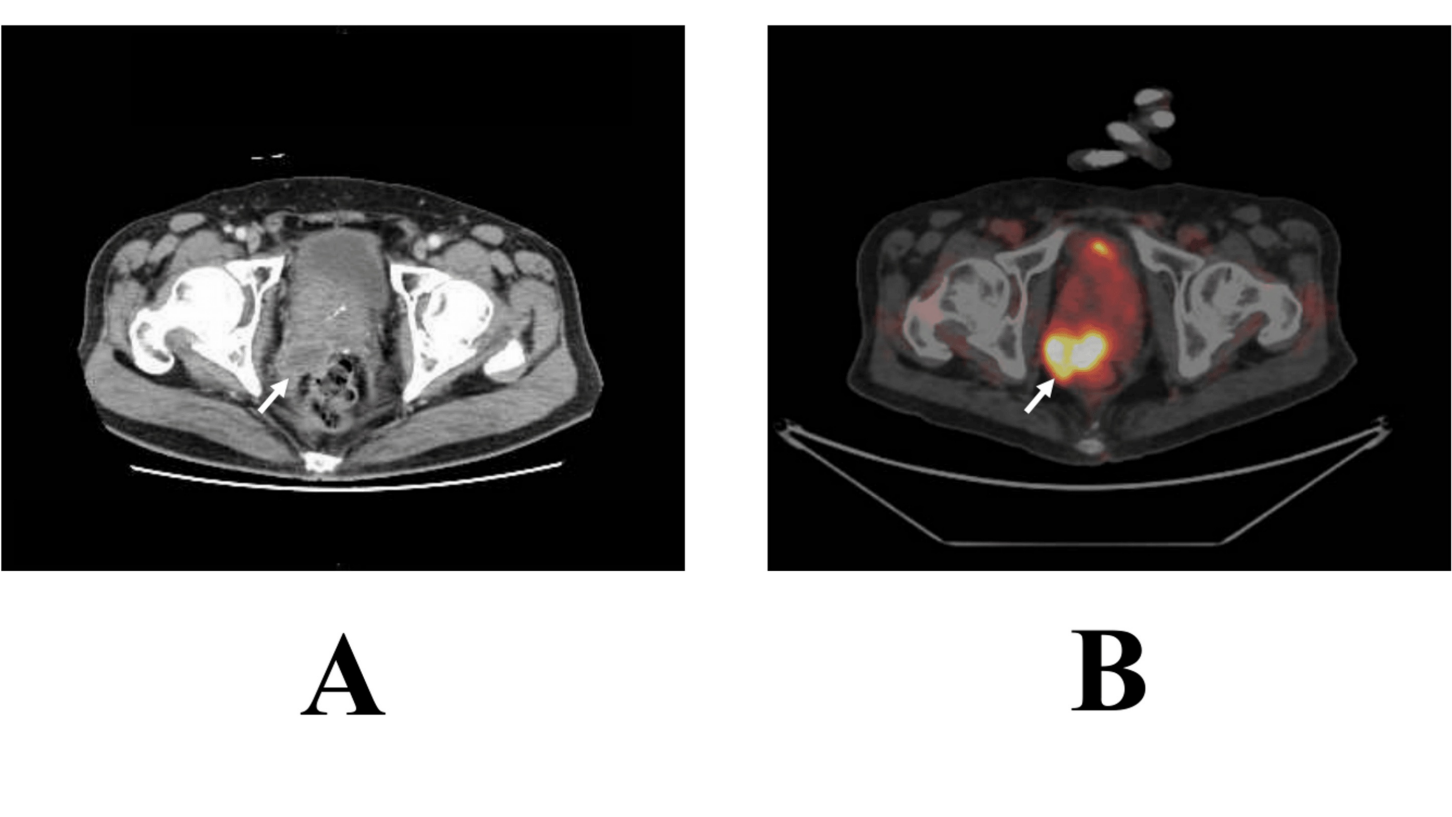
PET and CT findings A. The presumed metastatic tumor had a ring enhancement (arrow). B. PET showed a maximal standardized uptake value of 8.9 g/mL (arrow). PET, positron emission tomography; CT, computed tomography
